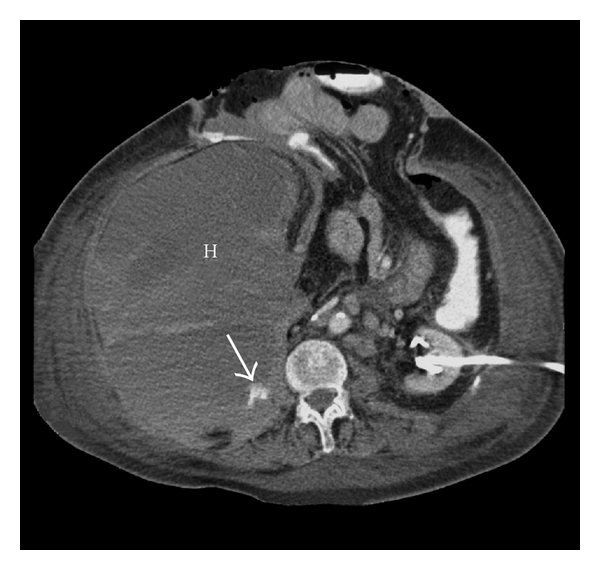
CT angiography demonstrating a large retroperitoneal hematoma (H) with evident mass effect and contrast extravasation (arrow).
